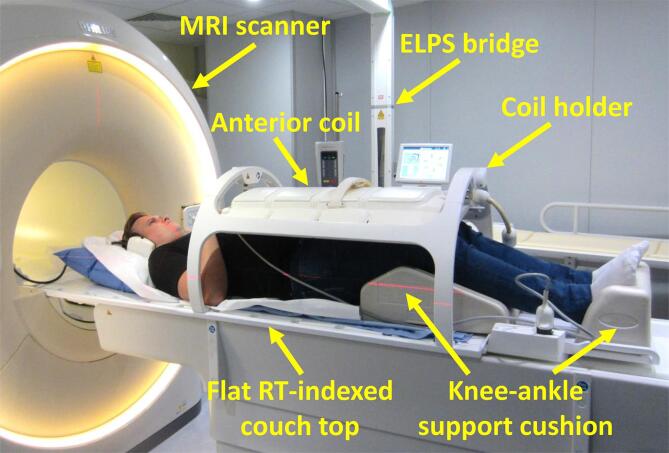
Patient setup on the magnetic resonance imaging (MRI) scanner used for the radiation therapy (RT) planning of prostate cancer: an external laser positioning system (ELPS), an anterior coil placed above the patient using a coil holder, a flat RT-indexed couch top and a knee-ankle support cushion.
